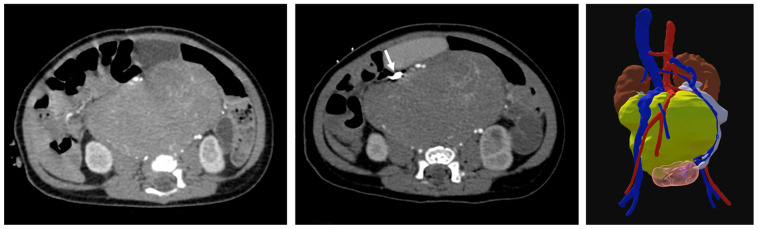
A 71-day-old boy with neuroblastoma centered at the aortic bifurcation. Initial pre-operative CT was obtained using an upper-extremity injection technique (left). The infrarenal IVC and iliac veins were not visualized. The exam was then repeated using a lower-extremity injection technique with excellent visualization of the IVC and extremity veins ((middle) arrow). Three-dimensional models were subsequently generated from the second scan for the purposes of surgical planning (right). Tumor shown in yellow, kidneys in orange, urinary bladder in pink, ureters in white, arteries in red, and veins in blue.
